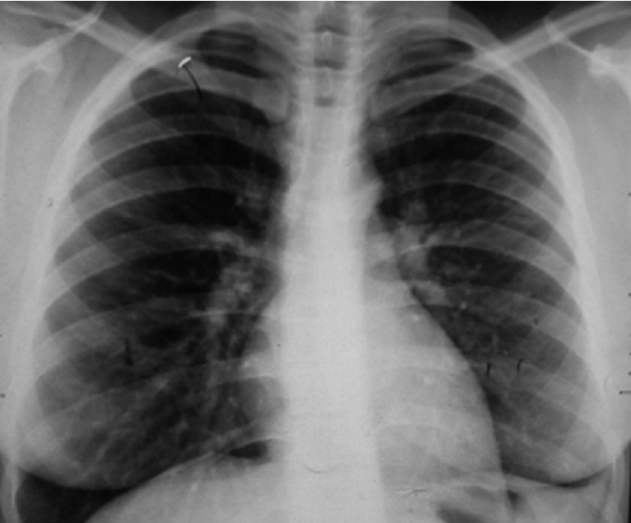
Radiographie thoracique de face: clartés finement cerclés au niveau basithoracique gauche: ascension de la coupole diaphragmatique gauche en rapport avec la lobectomie
